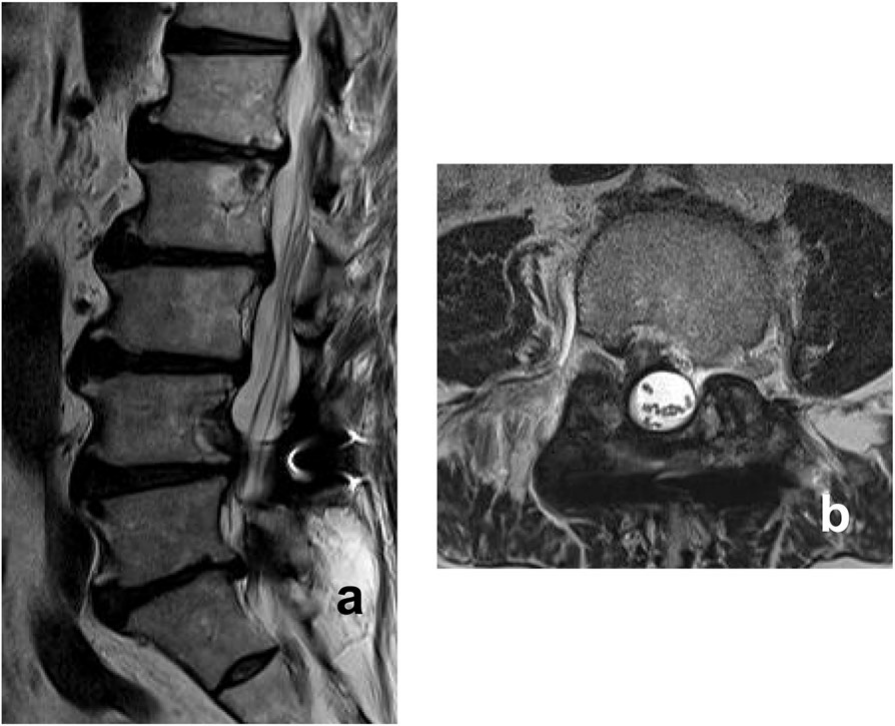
Postoperative magnetic resonance imaging (MRI) findings. a T2-weighted MRI of the sagittal and (b) axial aspects at the L4 vertebral level show the disappearance of the epidural space-occupying lesion at 2 years postoperatively
